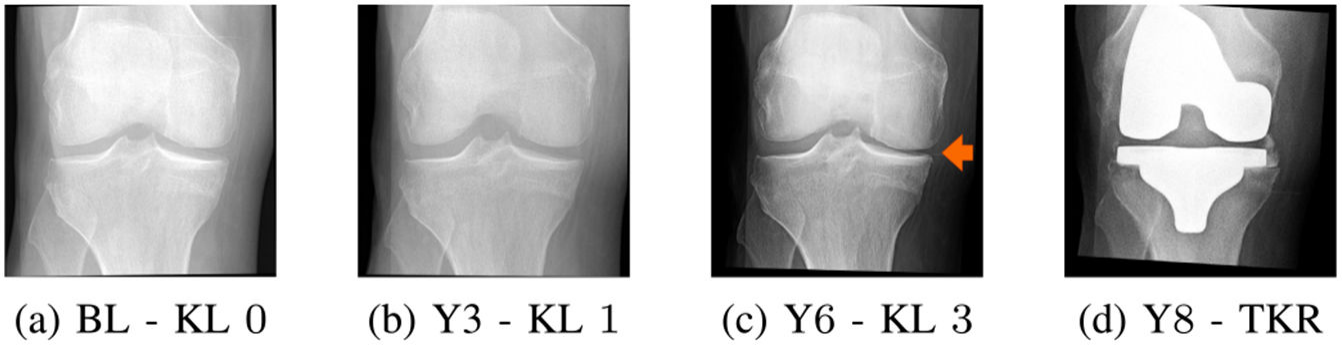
Radiographs of a patient with knee OA progressed in 8 years. The orange arrow indicates joint space narrowing. The disease progressed from Kellgren-Lawrence (KL) grade 0 at the baseline (BL) to 3 in 6 years. At the 8th year, the patient underwent a total knee replacement (TKR) surgery.
